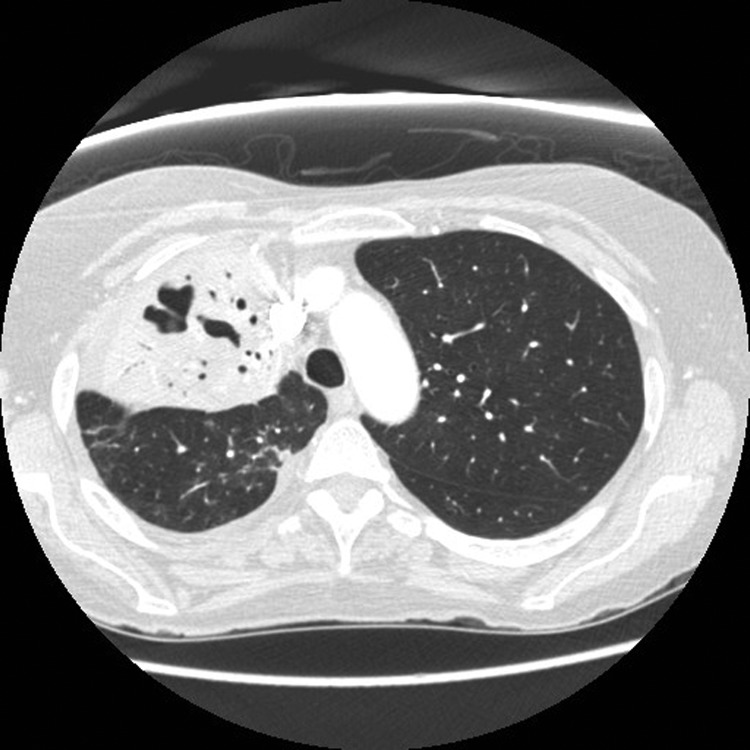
Results of repeat computed tomographic scan in a 67-year-old woman with *Legionella pneumophila* serotype 1 pneumonia 2 months after hospital admission. The scan shows reduction in the amount of consolidation and evolution of the lung cavity.
